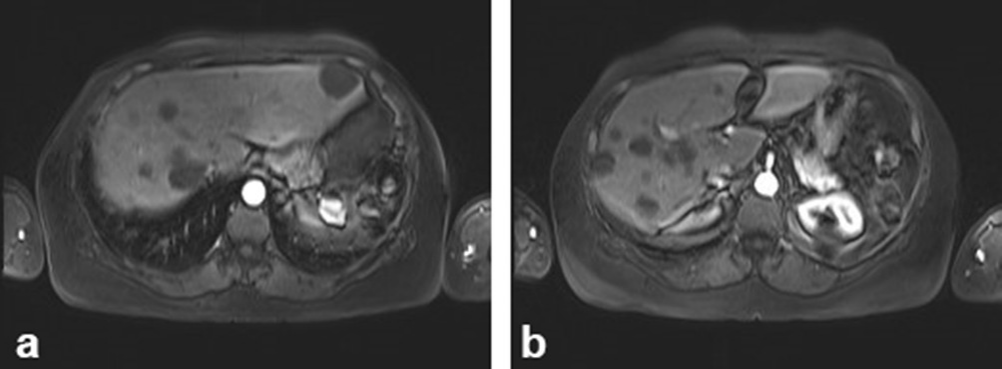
(a, b) MRI axial, arterial phase: multiple low-intensity lesions are identified that do not show contrast medium uptake.
